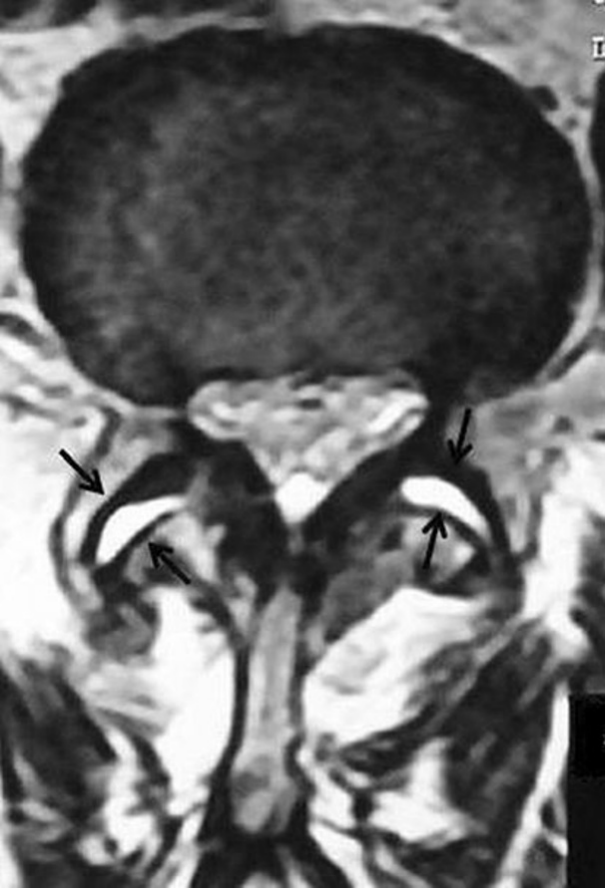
Same patient as in Fig. 1. Axial T2-weighted MRI shows “exaggerated” fluid in the facet joints at L4–L5 (*arrows*)
